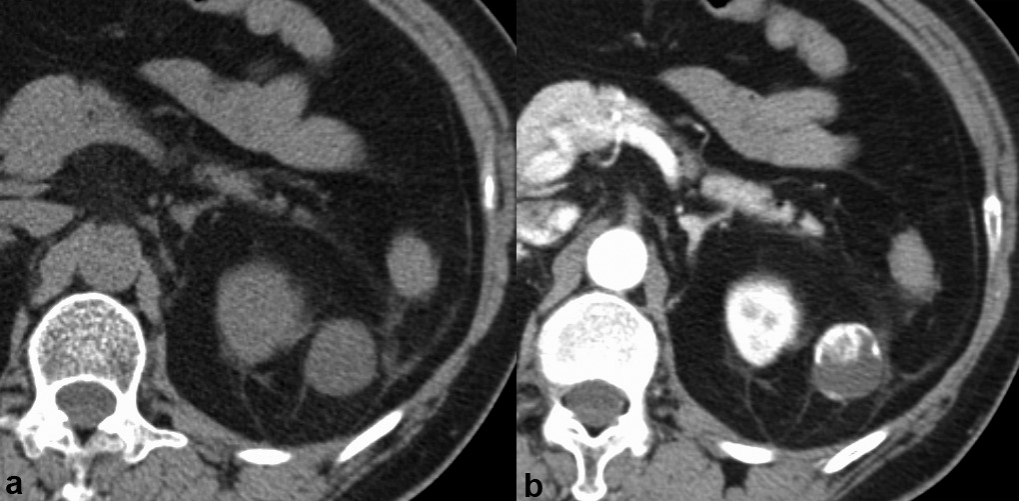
CT images of retroperitoneal anastomosing haemangioma. (a, b) The ventral side of the mass exhibited avid contrast enhancement equivalent to the renal cortex in the corticomedullary phase.
